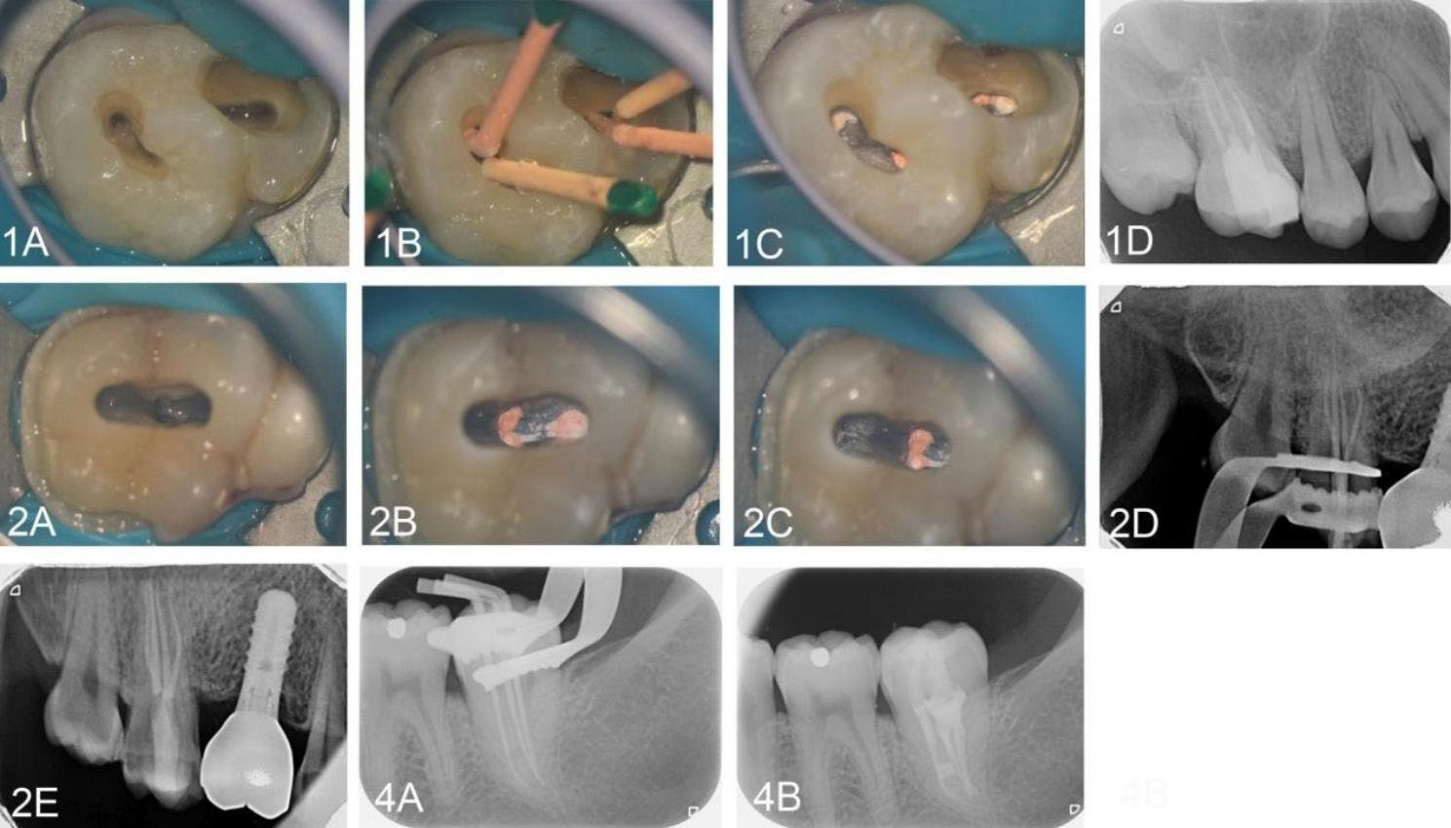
Representative intraoral photos of the diseased teeth during root canal therapy and radiograph of the teeth immediately on completion of endodontic treatment
